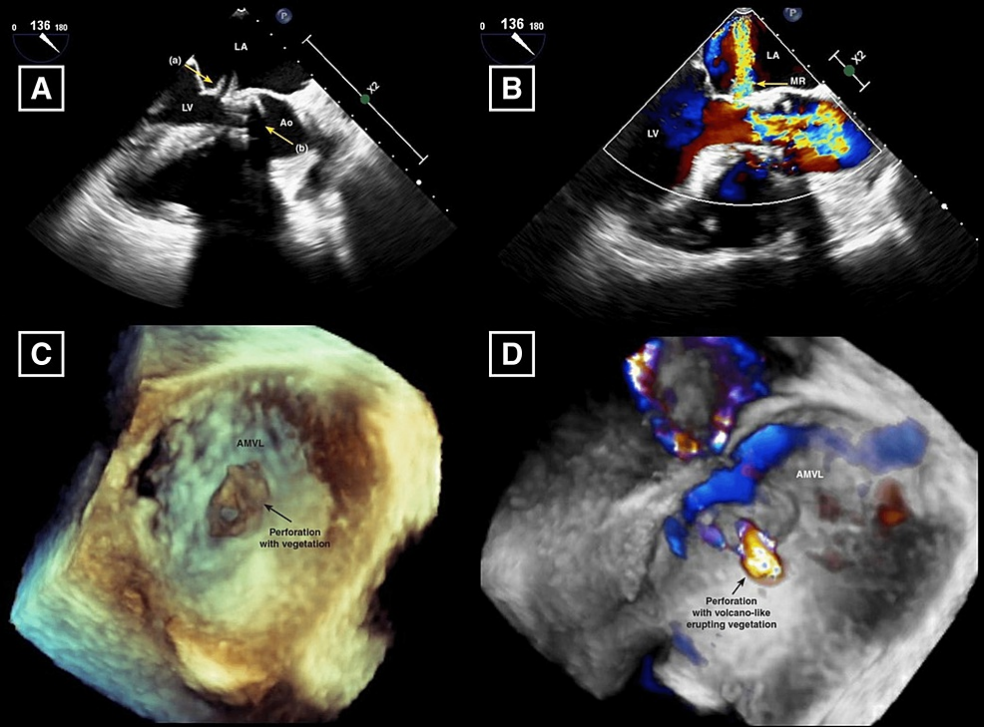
Transesophageal echocardiogram. A: 2D three-chamber view showing anterior mitral leaflet valve perforation with vegetation (yellow arrow A) along the TAVR (yellow arrow B). B: Doppler three-chamber view showing moderate to severe mitral regurgitation jet through the perforation. C: 3D view demonstrates anterior mitral leaflet valve (AMLV) perforation with attached vegetation. D: Doppler 3D view showing volcano-like vegetation coming out of AMLV perforation.
